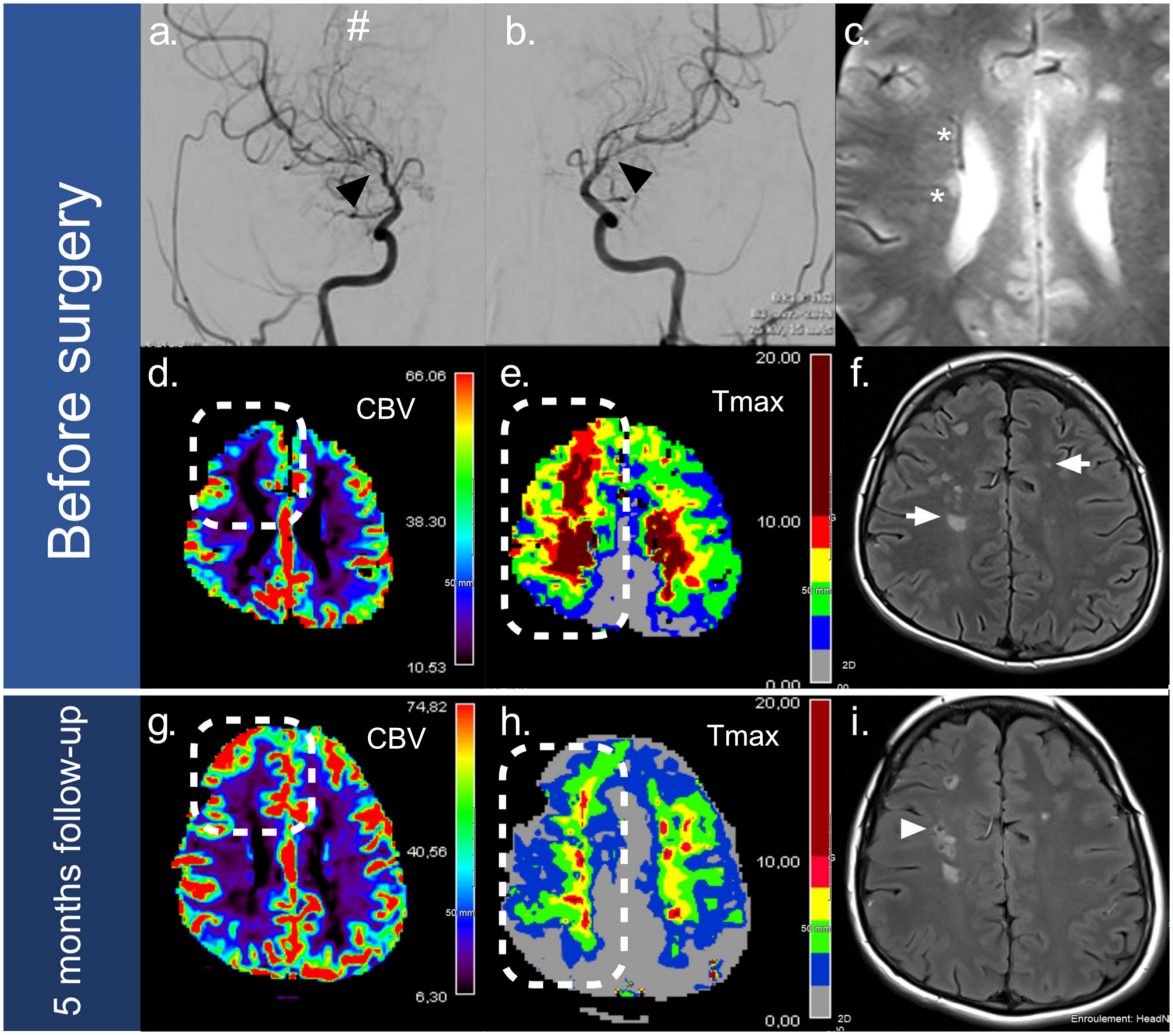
Imaging of moyamoya lesions and 5-month follow-up. Before surgery, moyamoya lesions were observed on the right (a) and left (b) internal carotid artery angiograms, revealing bilateral stenosis of the internal carotid arteries termination (black arrows), with moyamoya collaterals (#). Magnetic resonance imaging (c–f) found asymmetric enlargement of the medullary veins in relation with cerebral hypoperfusion (*) visible on the T2-GE sequence (c), associated with bilateral watershed ischemic lesions on FLAIR (f) (white arrow). Perfusion imaging pointed out severe hypoperfusion in the right frontal junctional territory (squared dots) on the cerebral blood flow cartography (CBV) (d) and the TMAX cartography (e). A five-month follow-up scan (g–i) showed significant improvement of the left frontal lobe perfusion after surgery with: (1) an increase in cerebral blood flow (d vs. g) (squared dots), and (2) shrinkage of delayed perfusion areas on TMAX >6s (e vs. h) (rectangular dots). No recurrent ischemic lesions were observed after surgery. Preoperative ischemic lesions progressed into lacunar lesions (f vs. i) (white arrowhead).
